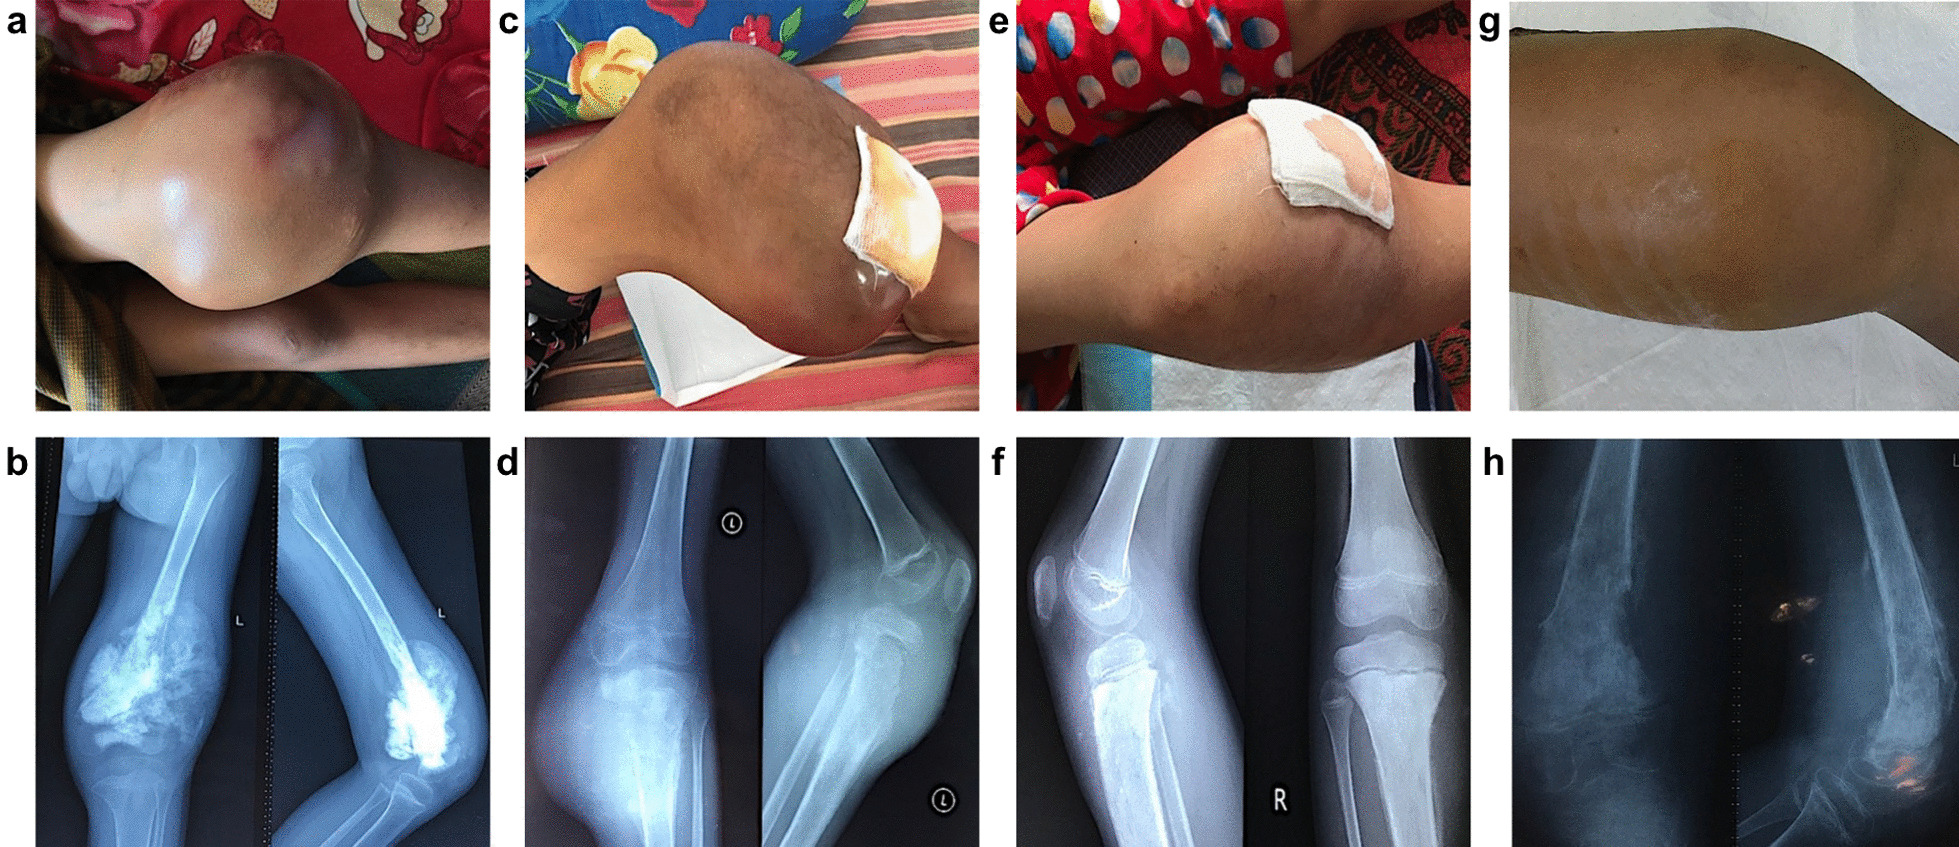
Clinical images and X-rays of patients 4–7. a and b Patient 4 with osteosarcoma of the left distal femur and corresponding X-ray; c and d Patient 5 with osteosarcoma of the left proximal tibia and corresponding X-ray; e and f Patient 6 with osteosarcoma of the right proximal tibia and corresponding X-ray; g and h Patient 7 with osteosarcoma of the distal femur and corresponding X-ray
